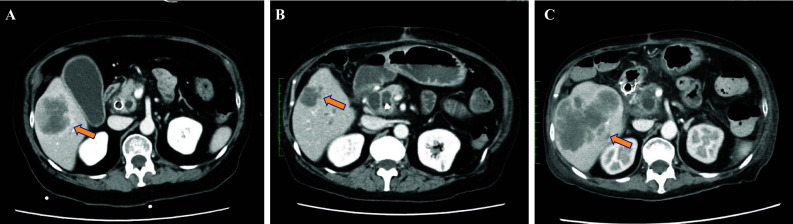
Abdominal computed tomography images during treatment with brigatinib. A, B Abdominal computed tomography showed the progression of liver metastases 2 years after the alectinib (A) and significant shrinkage of liver metastases 6 weeks after the brigatinb (B). C Abdominal computed tomography showed the progression of liver metastases 7 months after the brigatinb
